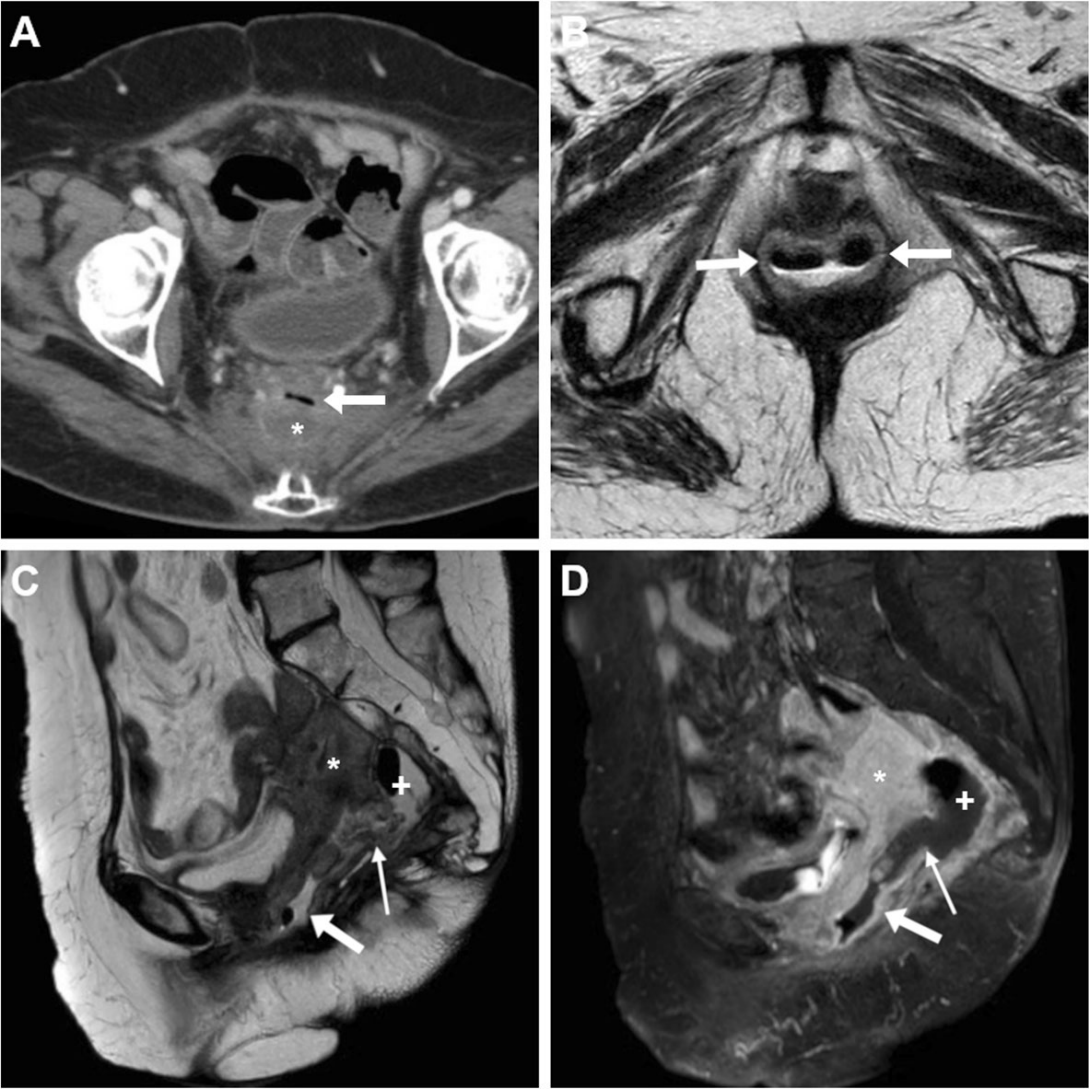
Neoplastic recto-VF, CT and MRI findings of VF-related vaginitis. In a 72-year-old with previous hysterectomy and resected rectal carcinoma, CT (a) shows local recurrence (*) indissociable from the air-filled vagina (arrows). Despite impossible gynaecological exploration, T2-weighted images (b, c) and fat-suppressed (FS) post-gadolinium T1-weighted (d) MRI images show increased solid tumour mass (*), air- and fluid-filled vagina (arrows) with circumferential wall thickening, oedematous T2 signal intensity and positive enhancement. The fluid-filled communication (thin arrows) between presacral air-fluid collection (+) and vagina is consistent with VF
